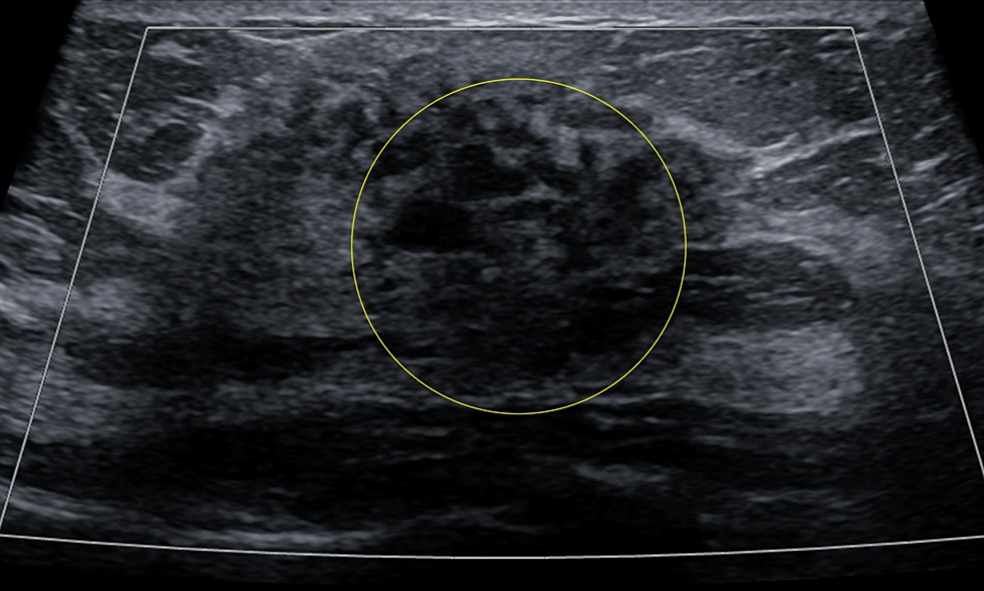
Transverse view ultrasound of the left breast showed a hypoechoic area at the 12 o’clock position with no internal vascularity (yellow circle).
